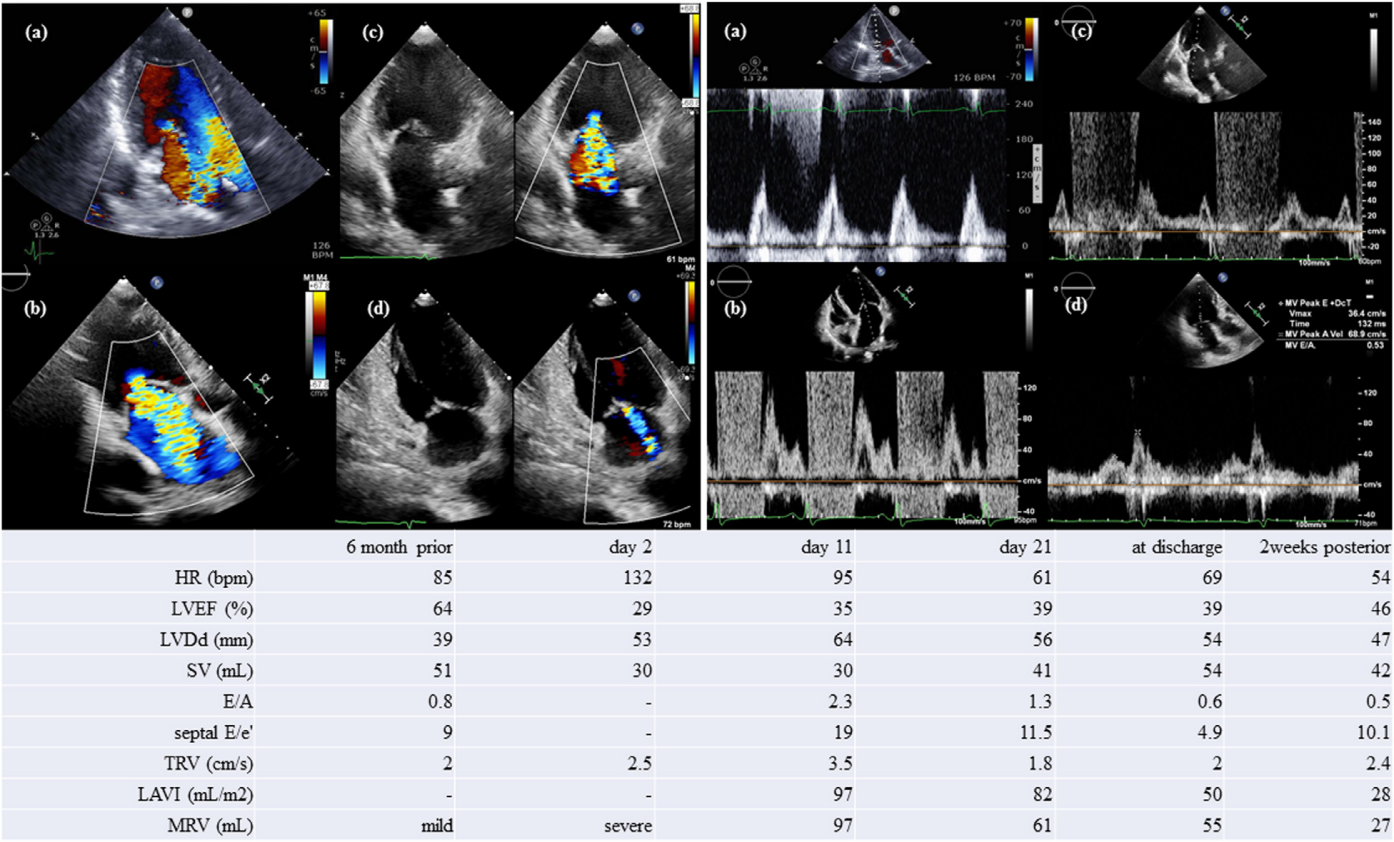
Transthoracic echocardiogram (TTE) changes. The chart shows the parameter of the TTE and her heart rate (HR) when she had had a TTE. The parameters include left ventricular ejection function (LVEF), left ventricular end-diastolic diameter (LVDd), stroke volume (SV) obtained by Doppler, early to late diastolic transmitral flow velocity (E/A), E to early diastolic mitral annular tissue velocity (E/e'), tricuspid regurgitant velocity (TRV), left atrial volume indexes (LAVI), and mitral regurgitation obtained by volumetric method (MRV). On day 2, mitral E and A waves fused due to tachycardia. The left panel shows color Doppler of mitral regurgitation. The right panel shows mitral inflow and mitral annular motion velocities. In both panels: (a) On day 2; (b) On day 11; (c) On day 21; (d) 2 weeks posterior to discharge.
